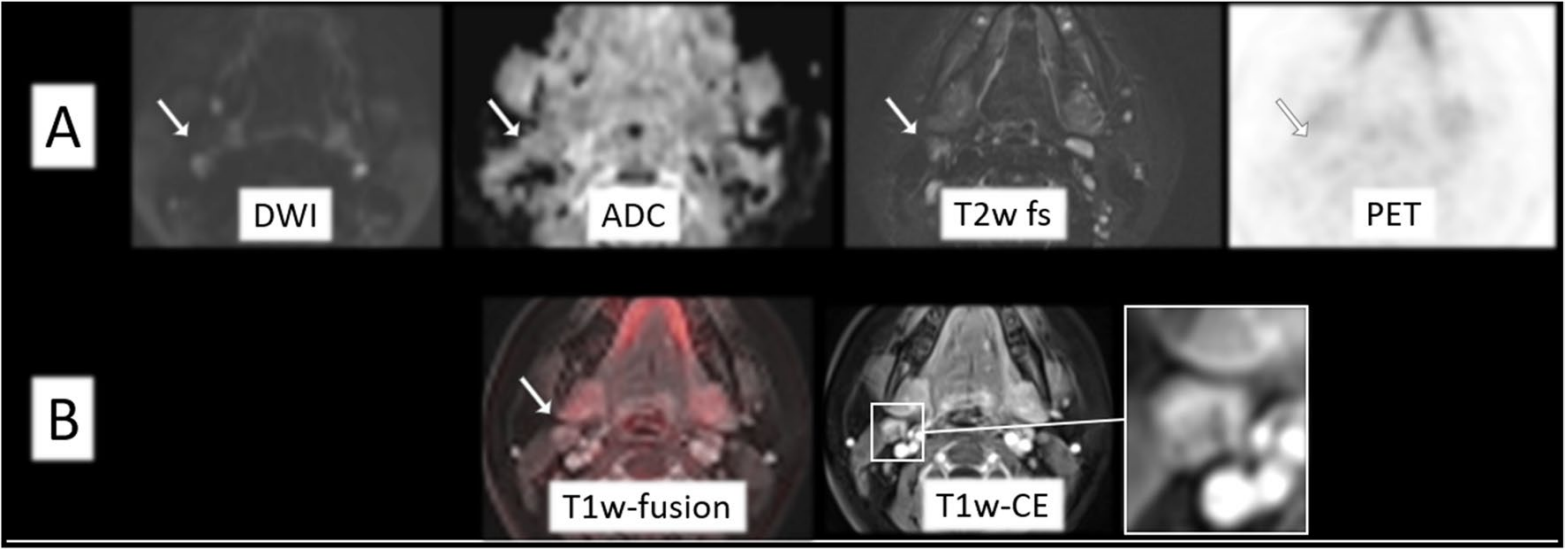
Example of a 13-year-old pediatric patient with missed active lymphoma disease at PET/MRI-1 (A) and PET/MRI-2 (A, B) according to the standard of reference. No visual [^18^F]FDG uptake (SUVmax: 2.0) was seen. Non-pathological appearance of the lymph node right cervical with borderline size (13 mm). Minimal contrast agent accumulation (white frame) within the lymph node in the T1-weighted (w) contrast-enhanced (CE) sequence. However, in synopsis of the images, this did not change the evaluation
